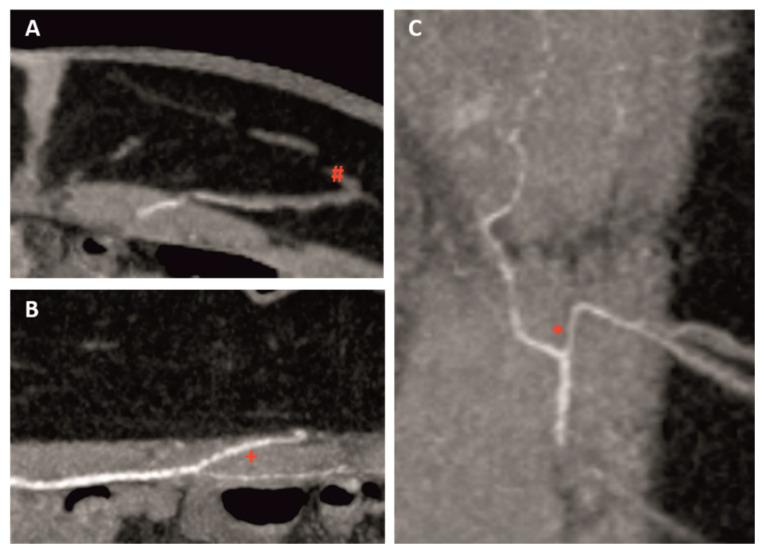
Perforator and DIEA mapping using computed tomographic angiography (CTA) in transversal (A), sagittal, (B) and coronar (C) view (#: connection to SIEV, +: intramuscular course, *: branching of DIEA).
